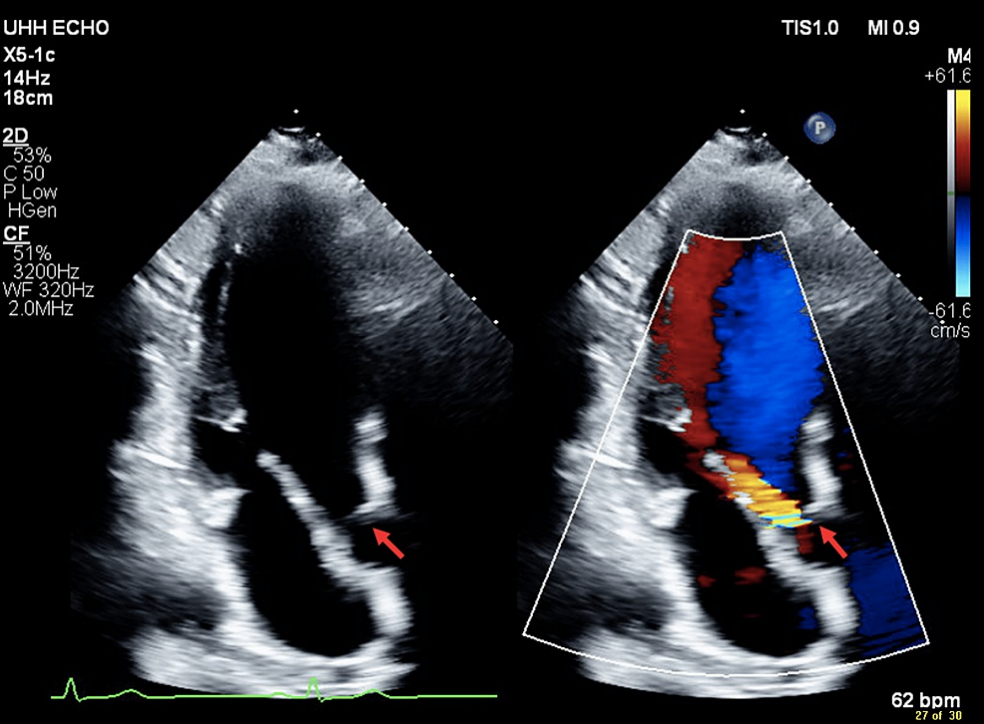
Transthoracic echocardiogram with and without color Doppler demonstrating the presence of a subaortic membrane causing regurgitant blood flow through the aortic valve.
